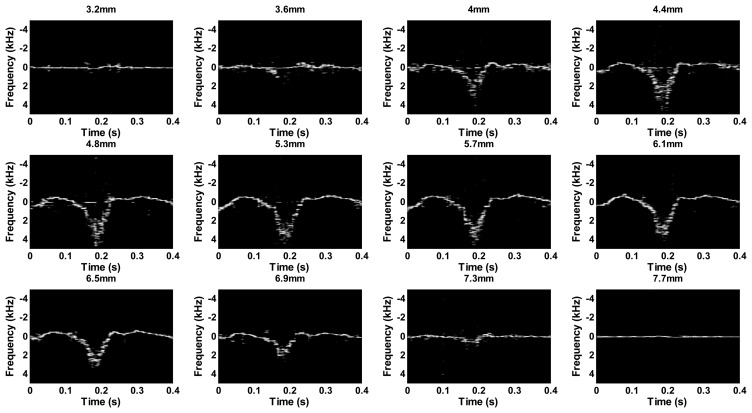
Sonogram of multigate PW Doppler with twelve interrogation gates.
